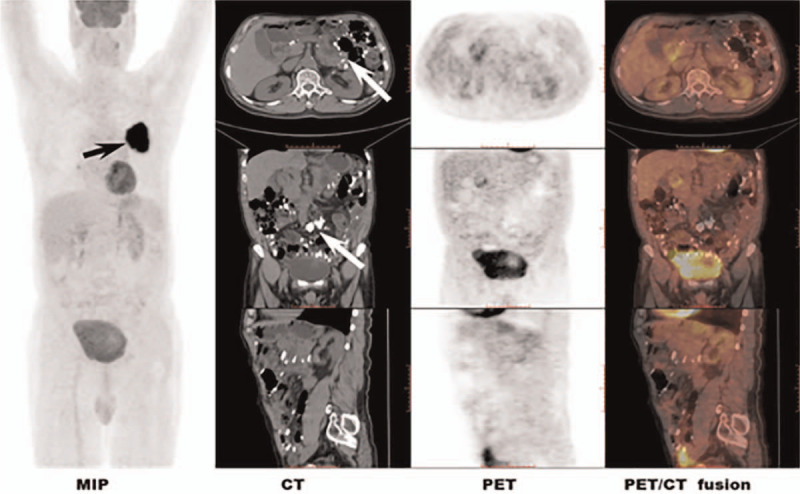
The MIP image demonstrates an irregular mass in the left upper lung field, exhibiting intense FDG uptake (black arrow). The abdominal CT reveal diffuse, amorphous calcifications in the greater omentum, mesentery of small bowel and colon (white arrow), with peritoneum thickening moderately. There is no apparent FDG-avidity in the corresponding PET images, with SUVmax of 1.0. SUV_max_ = the maximum of standard uptake value.
